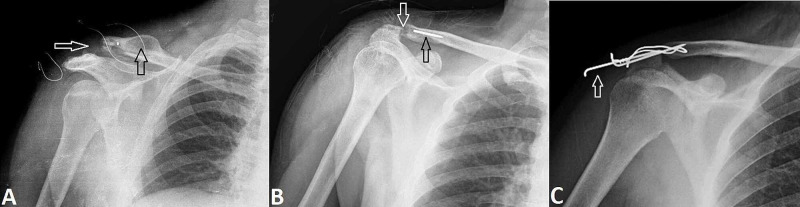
Radiographic images of complications Anteroposterior radiographic images of the shoulder show (A) residual dislocation (white arrow), osteolysis in the distal of the clavicle, and hypertrophic bone formation (black arrow), (B) distal clavicular osteolysis (white arrow) and Kirschner-wire breakage (black arrow), and (C) Kirschner-wire migration (white arrow).
